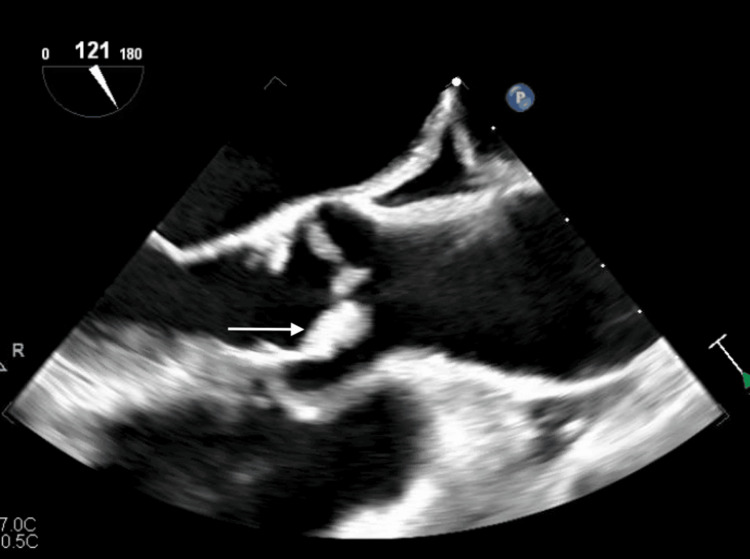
TEE image showing thickening of the aortic valve leaflets. The white arrow shows thickening of the aortic valve leaflets. TEE, transesophageal echocardiogram
